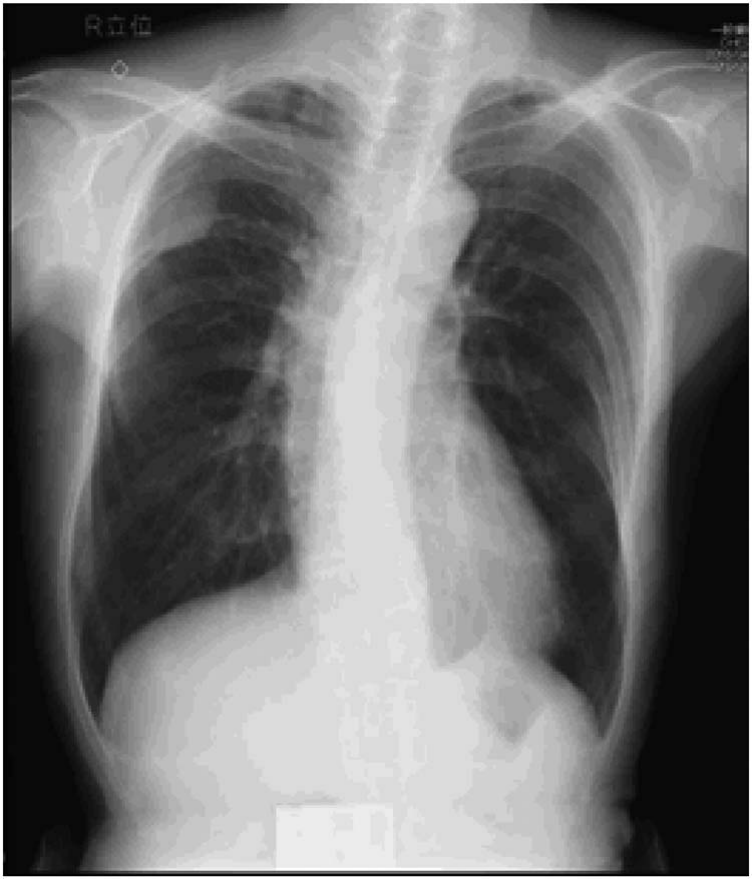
Preoperative chest X-ray: tumor shadow in the right upper lung field.
